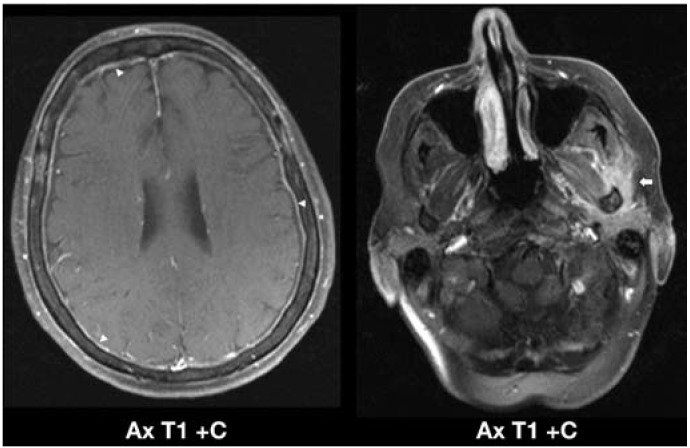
Meningeal infiltration related to AML in a previously healthy 69-year-old woman. Axial post-contrast T1-weighted images with fat saturation (left image) demonstrate marked pachymeningeal enhancement (arrowheads), most consistent with leukemic infiltration of the brain meninges. Note the marked ill-defined enhancement of the left infratemporal fossa (right image) surrounding the left masticator and deep parotid spaces (arrow), corresponding to extra-cranial leukemic myeloid sarcoma of the soft tissues of the left neck. Complete resolution of the leukemic infiltration within the deep left neck was observed after successful AML induction chemotherapy on follow-up imaging (not shown). Ax, axial; C+, post-contrast.
